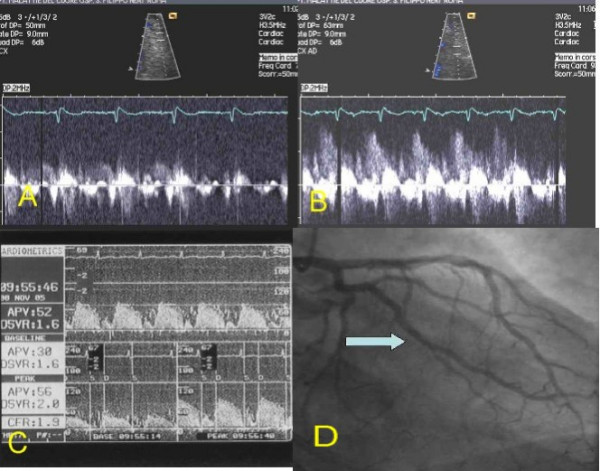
Patients 5. A: TTDE before adenosine. B: TTDE after adenosine. C: DW recording. D: coronary angiography with site of DW recording (arrow). DW: Doppler-wire. TTDE: transthoracic Doppler echocardiography.
